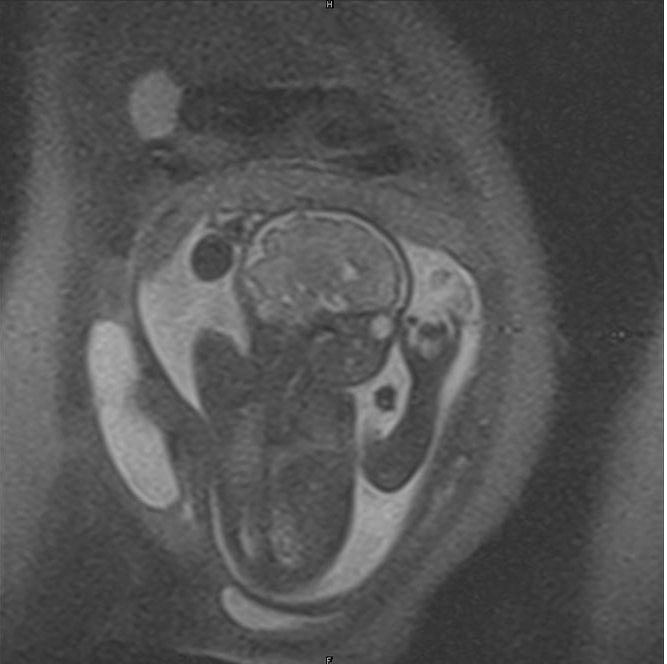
Showing MRI image of ovarian cyst with fetus in utero.
